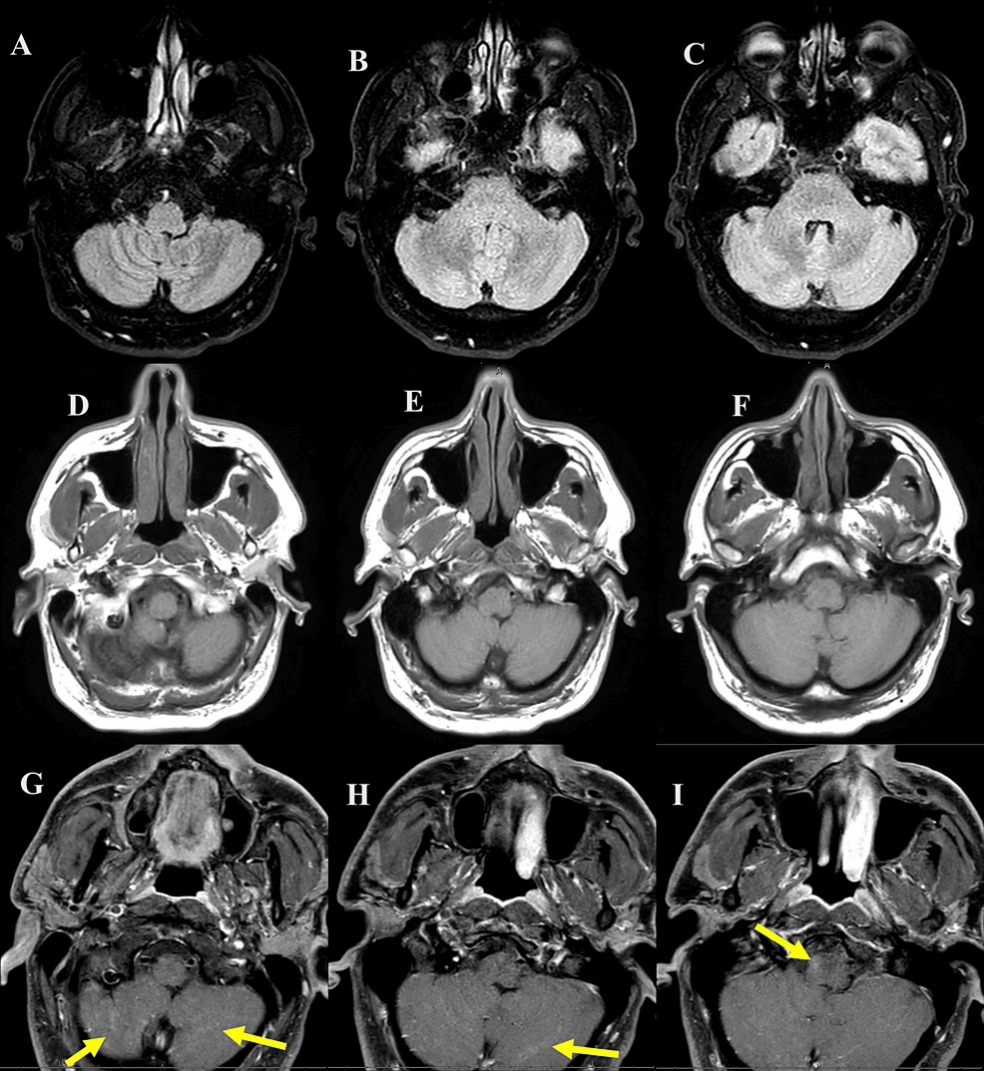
Axial FLAIR (A-C), axial T1 pre- (D-F), and post-contrast (G-I) brain magnetic resonance imaging (MRI) from day 2 of hospitalization. The imaging shows multiple tiny linear and punctate foci of subtle enhancement in the cerebellum especially inferiorly (possibly representing a small foci of leptomeningeal enhancement) and subtle punctate enhancement in the pons and medulla (yellow arrows). FLAIR: Fluid-attenuated inversion recovery
